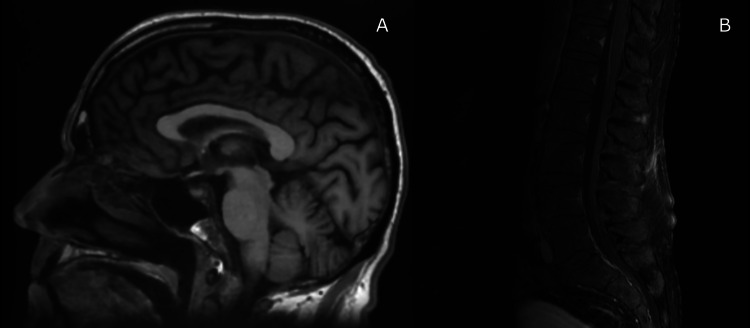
A) MRI of brain revealing normal parenchyma without masses. B) MRI of spine without cord compression or nerve root thickening. Questionable mild pial enhancement present.
